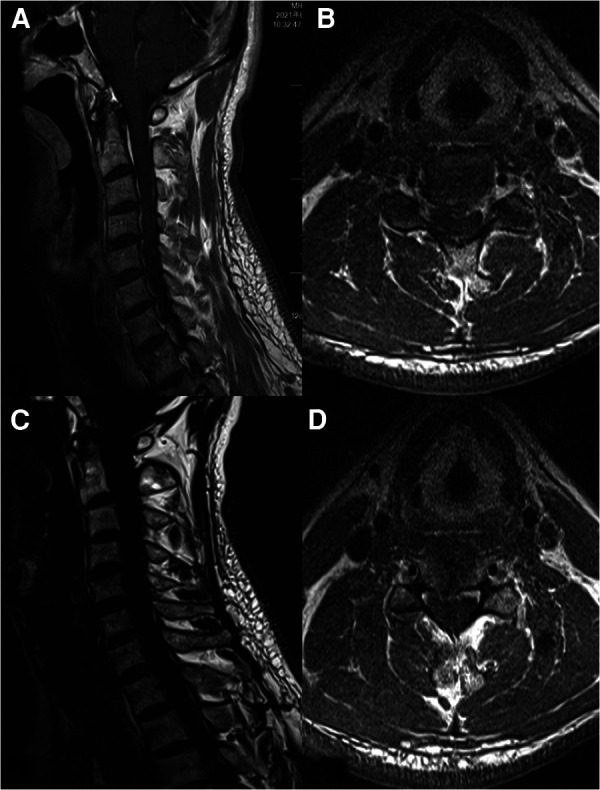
(A) Preoperative sagittal enhancement MRI of the cervical spine. (B) Preoperative C3/4 cross-sectional enhanced MRI. (C) Fat suppression of preoperative sagittal enhancement MRI. (D) Preoperative C4/5 cross-sectional enhanced MRI.
